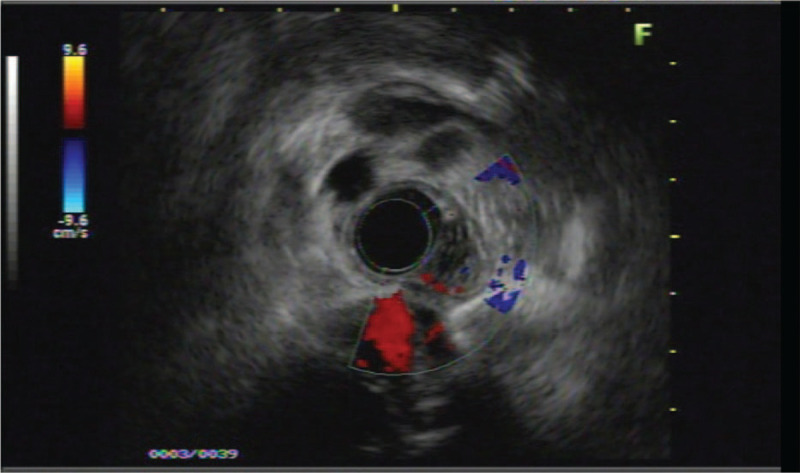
Endoscopic ultrasonography (EUS) using a 12-MHz probe revealed well-defined tumor with heterogenous echogenicity within the submucosa.
